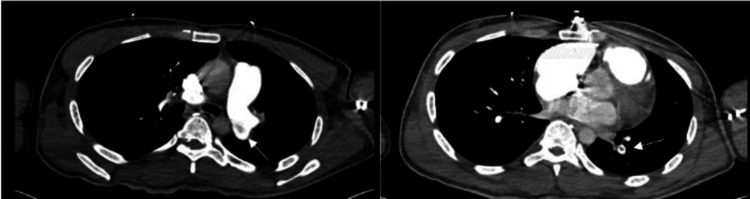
The CTA of the chest reveals pulmonary filling defect within the left main pulmonary artery. The white arrow points at the pulmonary embolism. CTA: Computed tomography angiogram
